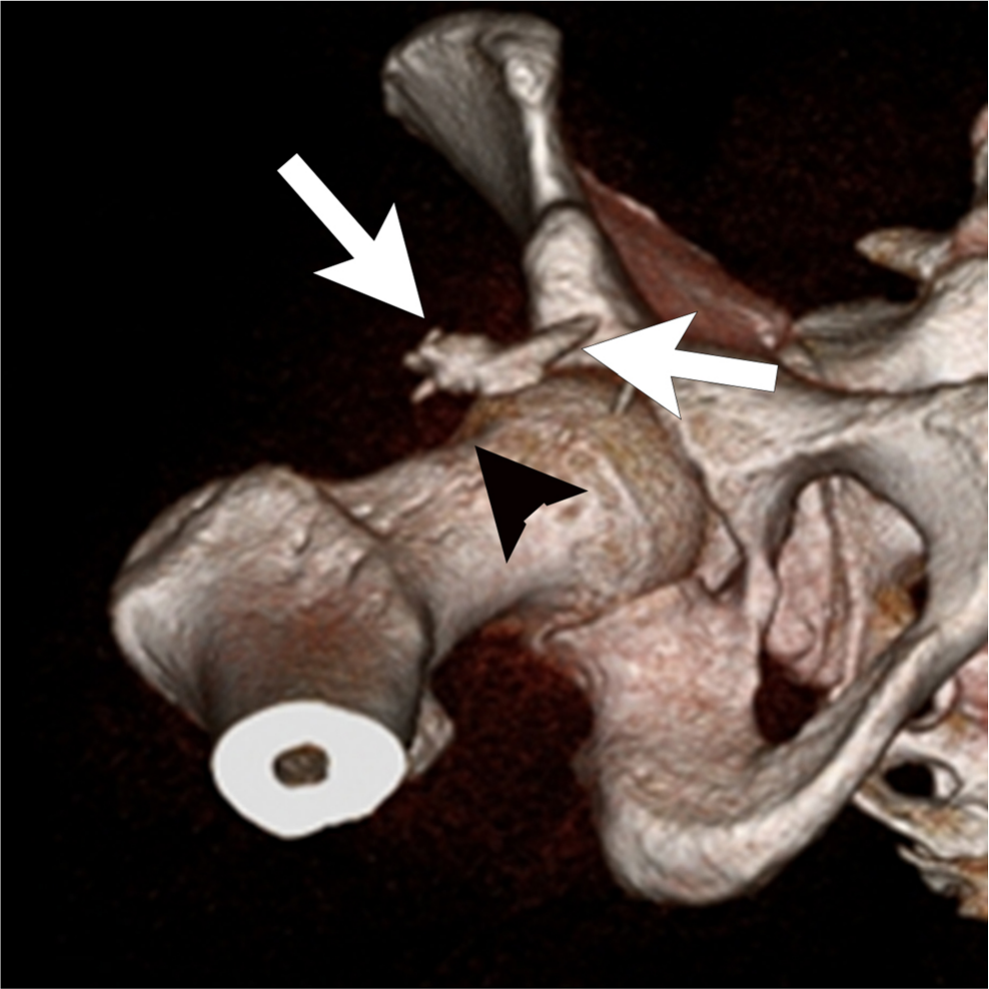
A 25-year-old man with a mild limitation of motion after arthroscopic resection of a cam lesion. 3D CT shows a sheet of heterotopic ossification (*arrows*) anterior to the femoral osteochondroplasty site (*arrowhead*)
